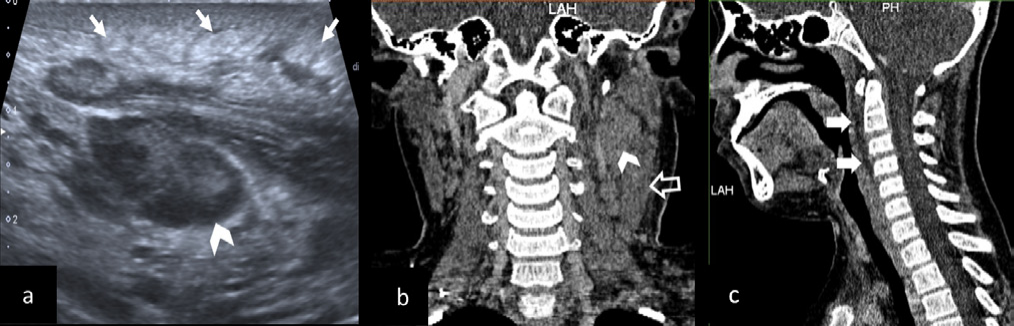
Imaging findings of a deep neck infection in a 10-year-old boy who presented with fever and swelling in the neck. (a) Ultrasonography image shows lymph nodes (arrow head) and edema of the skin-subcutaneous soft tissue planes (white arrows) (b). Coronal CT image shows lymph nodes (arrow head) and thickened sternocleidomastoid muscle (open arrow) (c). Sagittal CT image shows retropharyngeal and prevertebral edema (white arrows).
